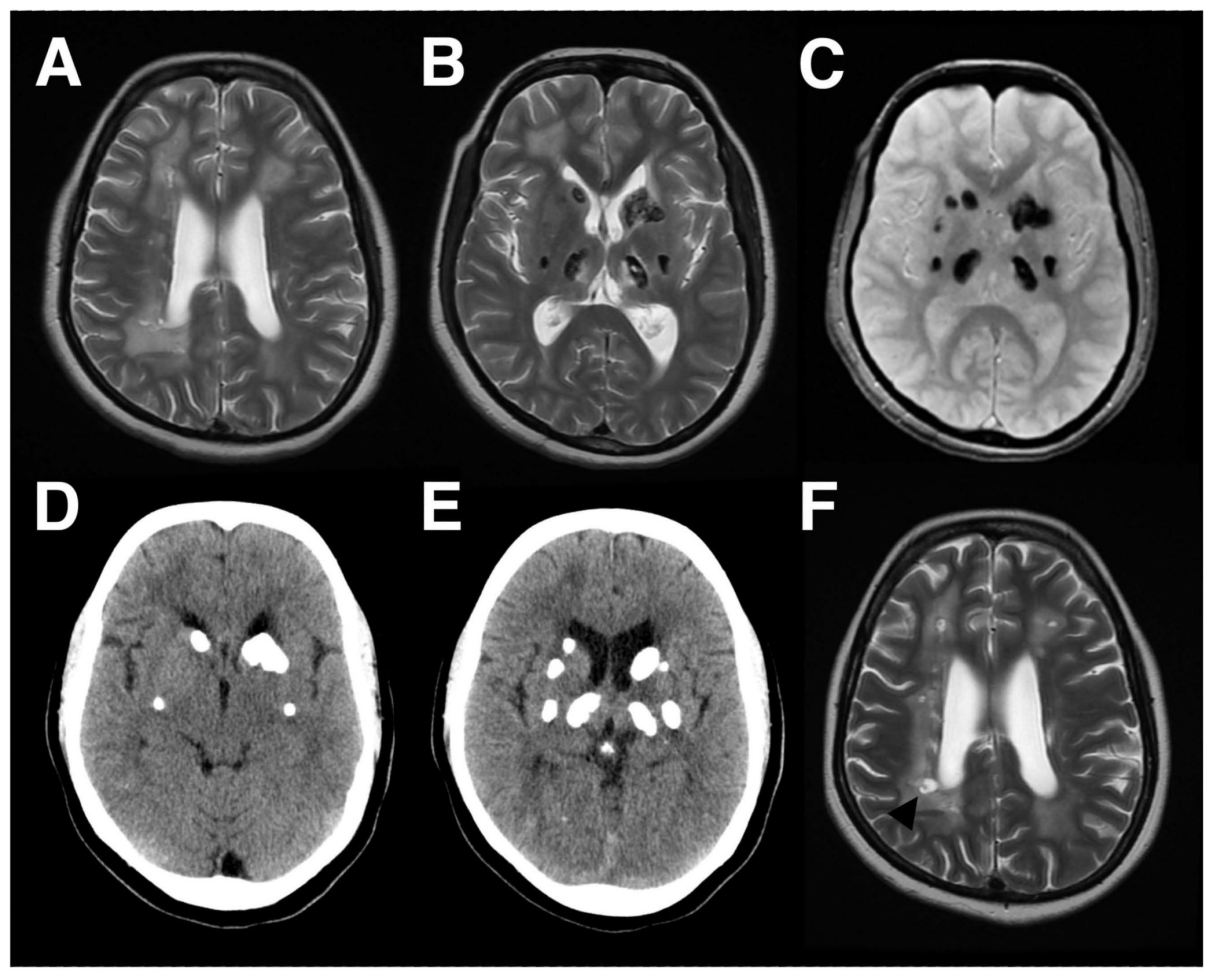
(A,B) Baseline Axial T2 Magnetic resonance (MR) of head shows asymmetric leukoencephalopathy with multifocal low signal areas in basal ganglia and thalami that bloom on Gradient echo (C);(D,E) Computed tomogram confirm corresponding extensive multifocal calcification ; (F) Follow-up axial T2-weighted MR shows development of periventricular cysts (black arrowhead).
